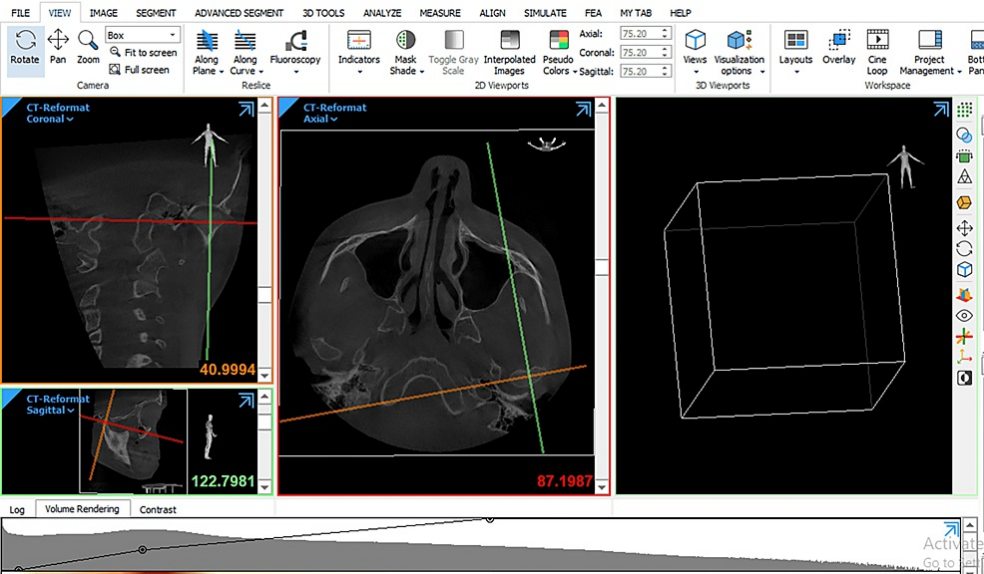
Cone-beam computed tomography (CBCT) images reorientation before reconstructing a 3D reproducible condyle model.
